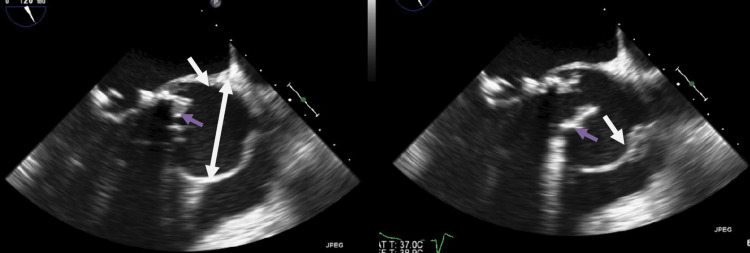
Transesophageal echocardiogram. Blue arrows show the prosthetic valve and white arrows show the walls of the aortic root pseudoaneurysm.
